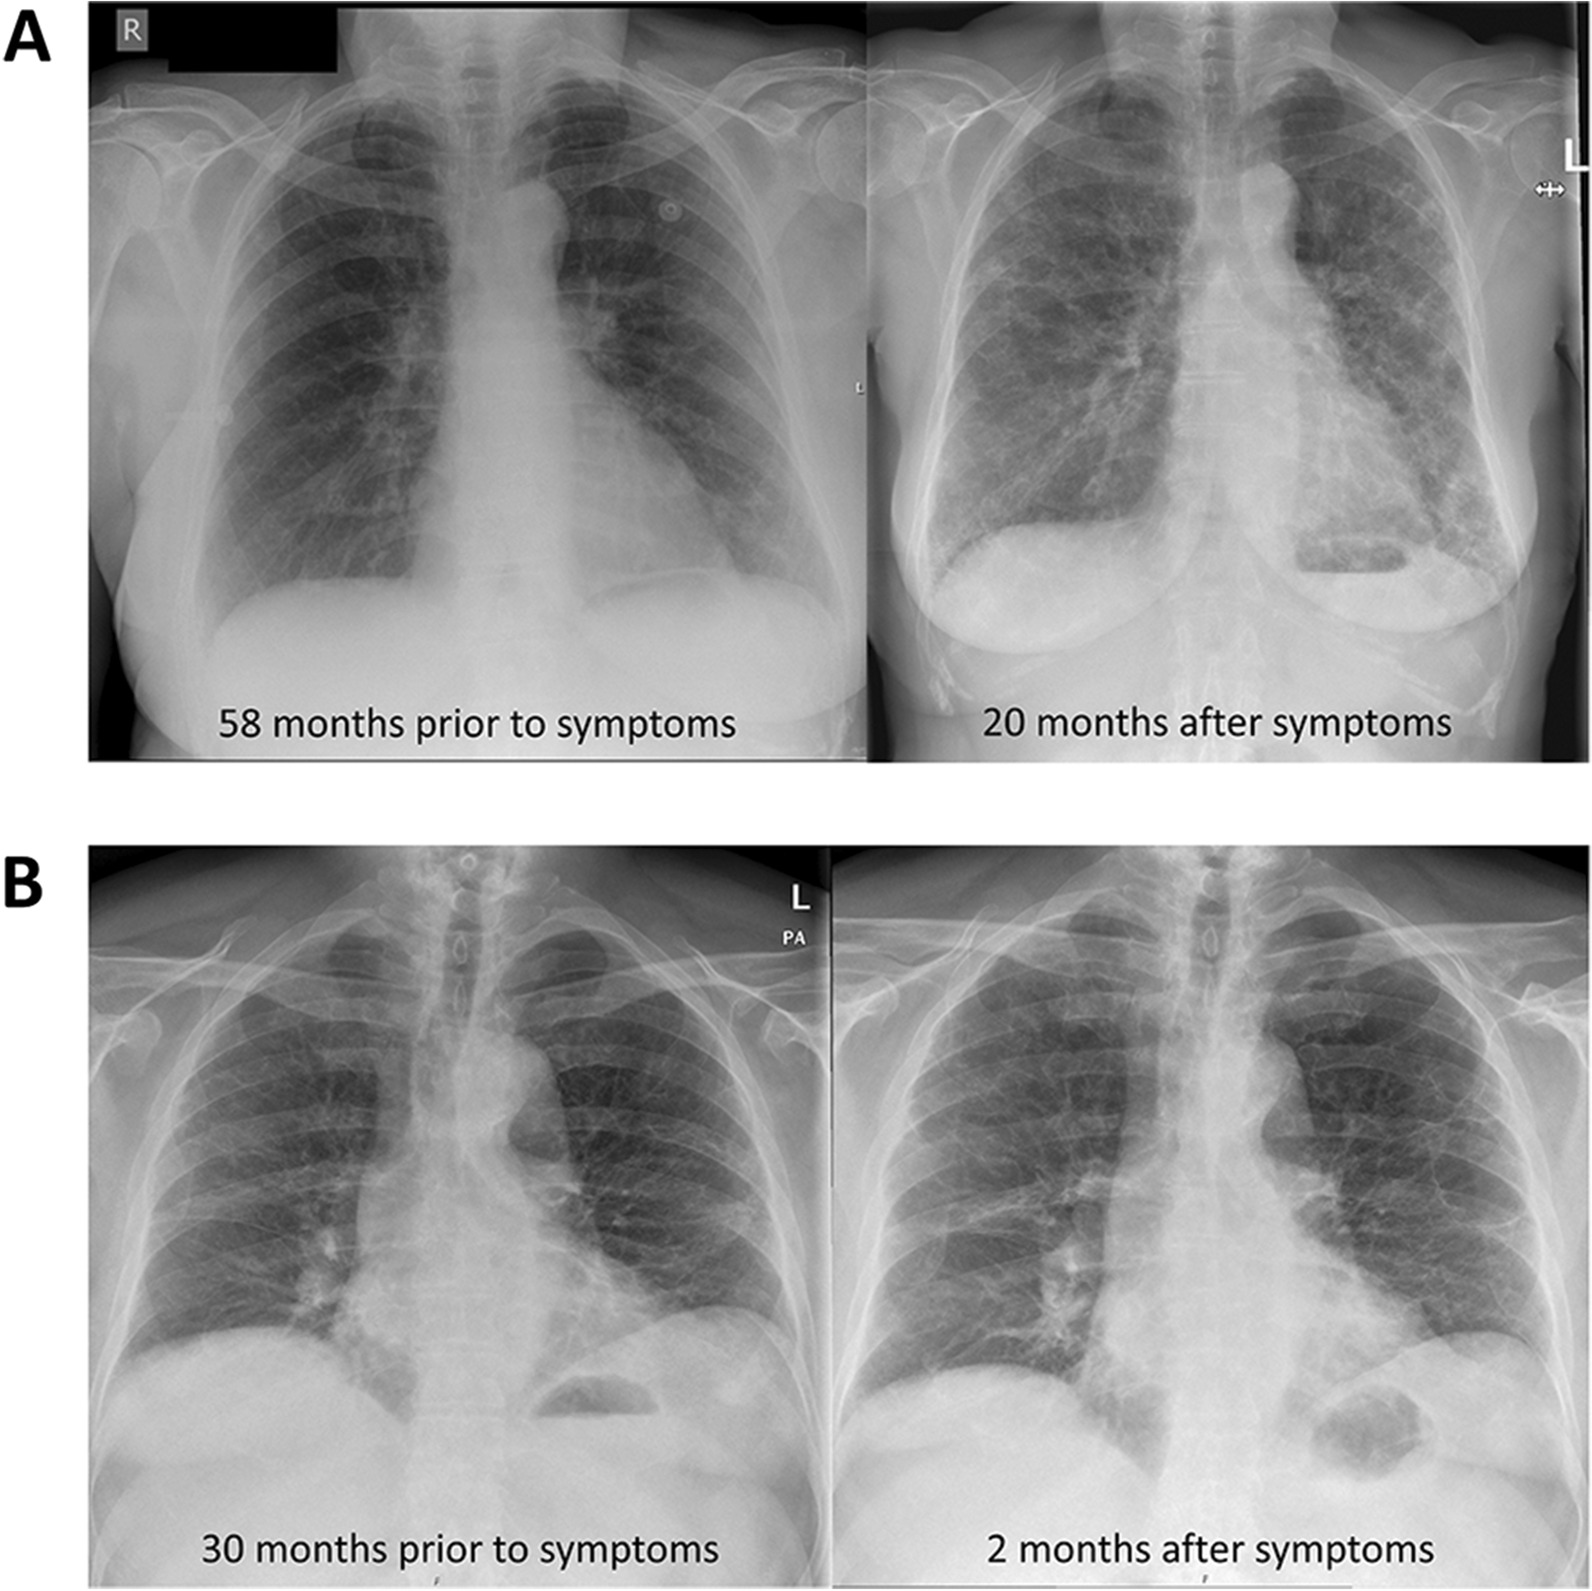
Examples of patients who had potential interstitial lung abnormalities on chest X-rays that were made prior to the start of symptoms. A patient who presented with temporary dyspnoea after a wasp sting 58 months prior to developing a cough and exertional dyspnoea. The chest X-ray at the time showed minimal reticular changes in the left lower lung. The chest X-ray that was made 20 months after symptoms started shows bilateral reticular changes in the lower fields, especially on the left. B patient who was evaluated for an episode of chest pain 30 months prior to developing a cough. The chest X-ray at the time showed bilateral reticular abnormalities. The chest X-ray that was made 2 months after symptoms started showed progressive reticular changes mainly in the lower fields
